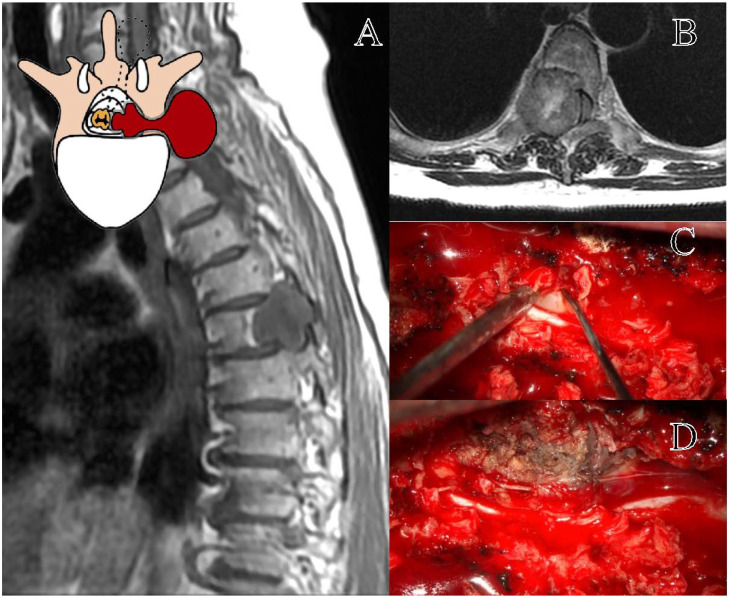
Representation of a T5–T6 schwannoma, Eden Type II. (A) Preoperative sagittal T1-weighted MRI with contrast, showing a lesion at the right T5–T6 level with both intradural-extramedullary and foraminal extension. (B) Axial MRI demonstrating the extradural and foraminal components of the lesion, with wide erosion and remodeling of the bone vertebral structure. (C) Intraoperative view following a laminectomy, exposing the tumor. (D) The transpedicular exposition of a tumor, with the demolition of the ipsilateral pedicle and articular facet.
